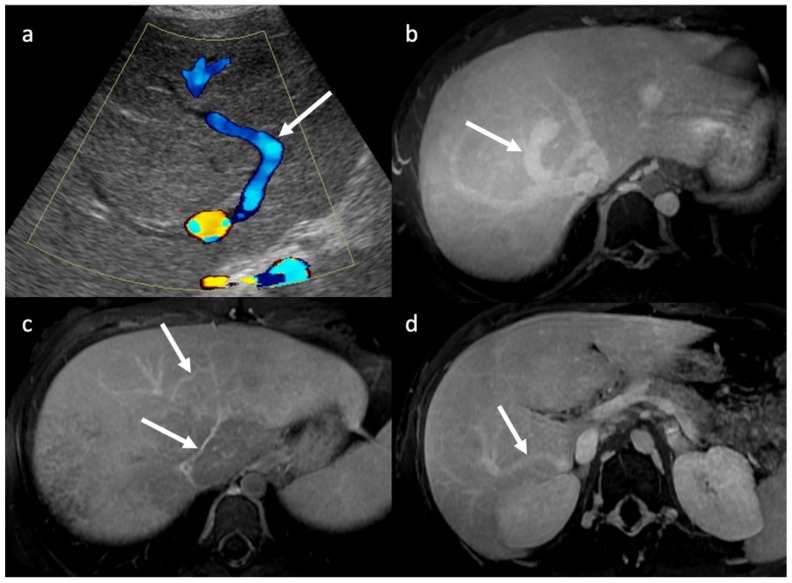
Color-doppler ultrasound (a) and axial 3 min delayed phase MR images (b–d) showing multiple veno-venous shunts (arrows) in different patients, as consequence of HV obstruction.
